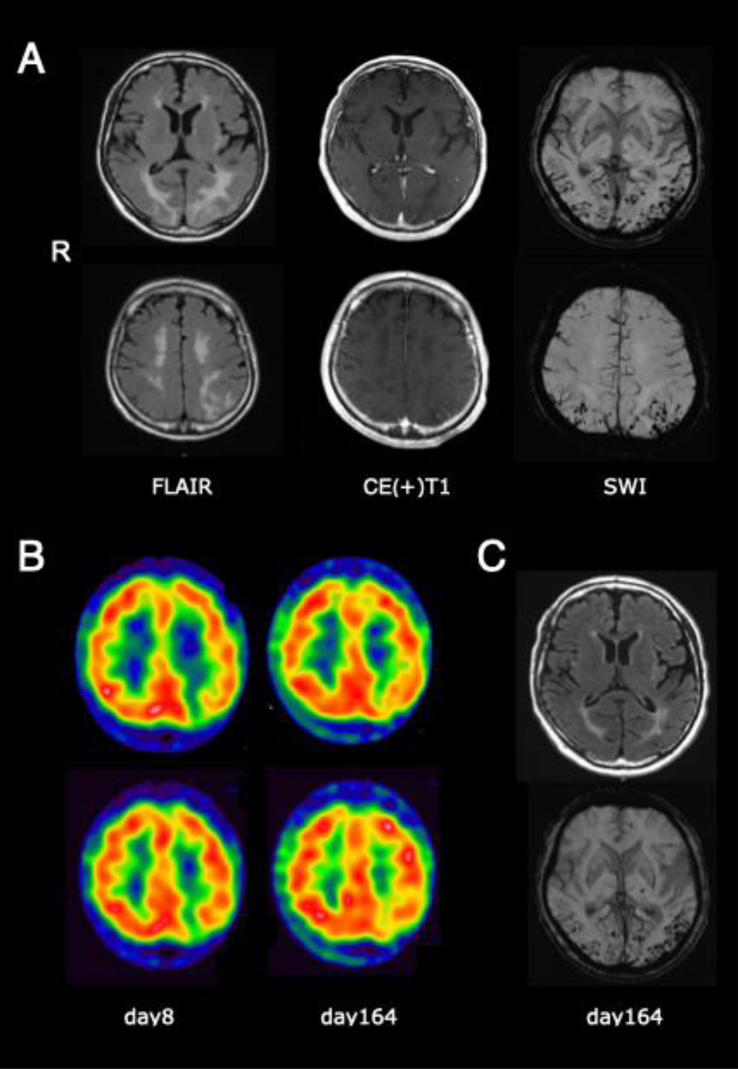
A. Head MRI fluid attenuated inversion recovery sequence (FLAIR) presents cerebral edema from the left occipital to parietal lobe. Gadolinium-enhanced contrasted effects of the superficial vessels in the same region. SWI shows multiple cortical MBs in the left occipital and parietal lobes. (B) 99mTc ECD SPECT. Reduced blood flow in the left occipital lobe at day 8, improvement seen at day 164. (C) MRI on day 164. Although FLAIR present increased signals improving under treatment progress, the MBs of SWI remain unchanged.
